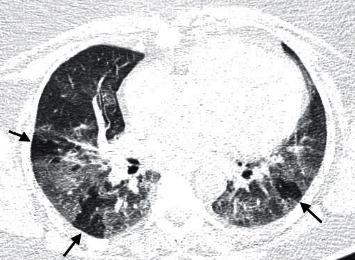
Chest CT in a lung window. Expiratory apnoea. An axial section at the level of the lower lobes highlights areas of air trapping (arrows).
